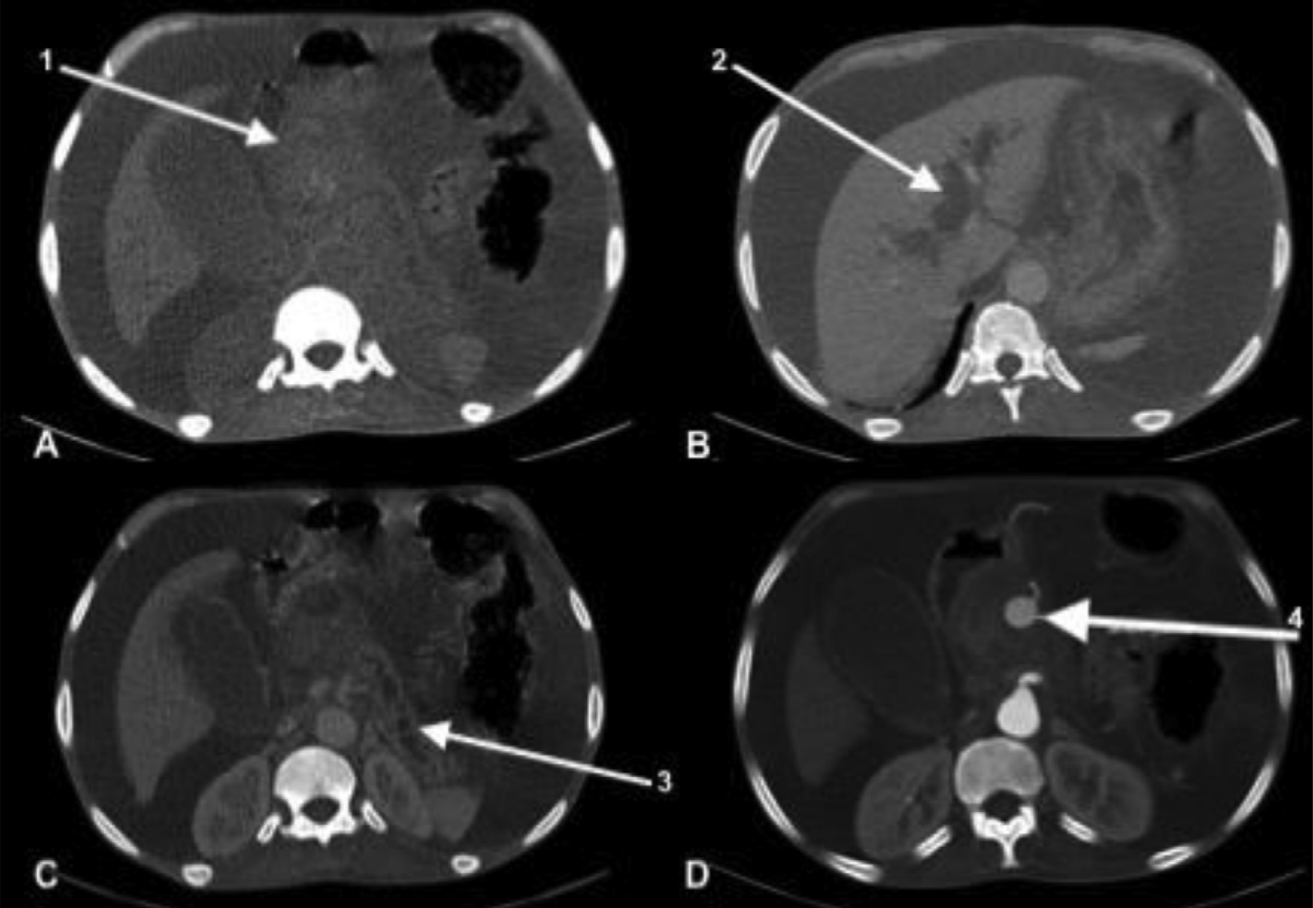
Abdominal CT scan showing in axial sections without injection (A) and with injection at the arterial and portal times (B, C and D) a pseudo-mass of the head of the pancreas (1) with dilatation of the intrahepatic bile ducts (2) and of the Wirsung duct (3). This pseudo-mass corresponds to a hematoma due to rupture of an aneurysm of the gastroduodenal artery (4)
